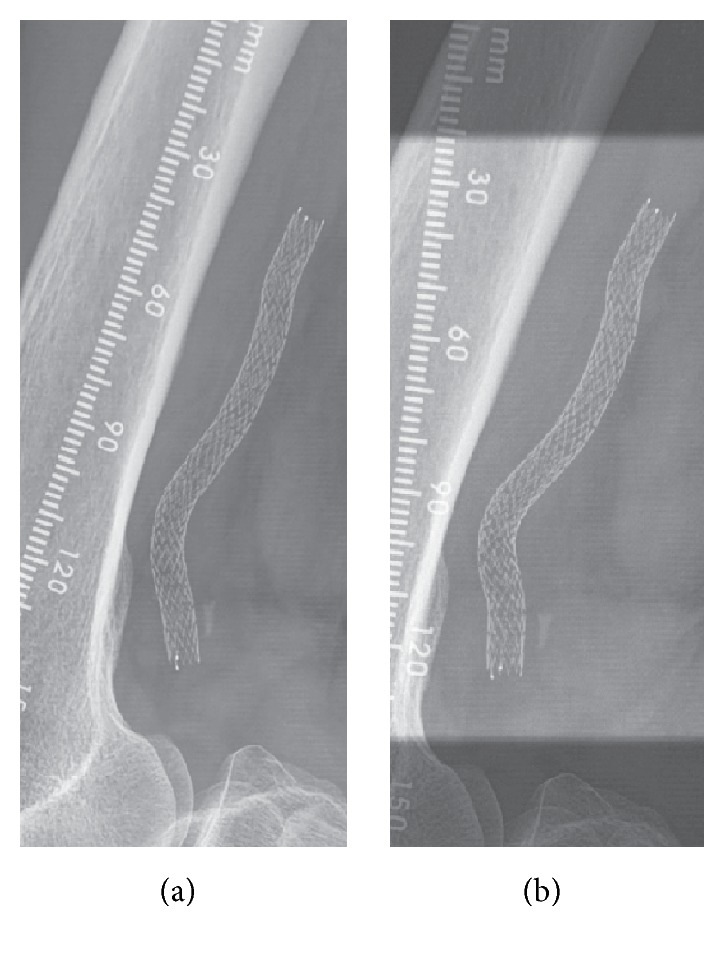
X-ray images of a BioMimics 3D stent in a femoropopliteal location in a patient with knee bent at 90 degrees, at 1 month (a) and 6 months (b) after implantation, showing sustained stent curvature over time.
